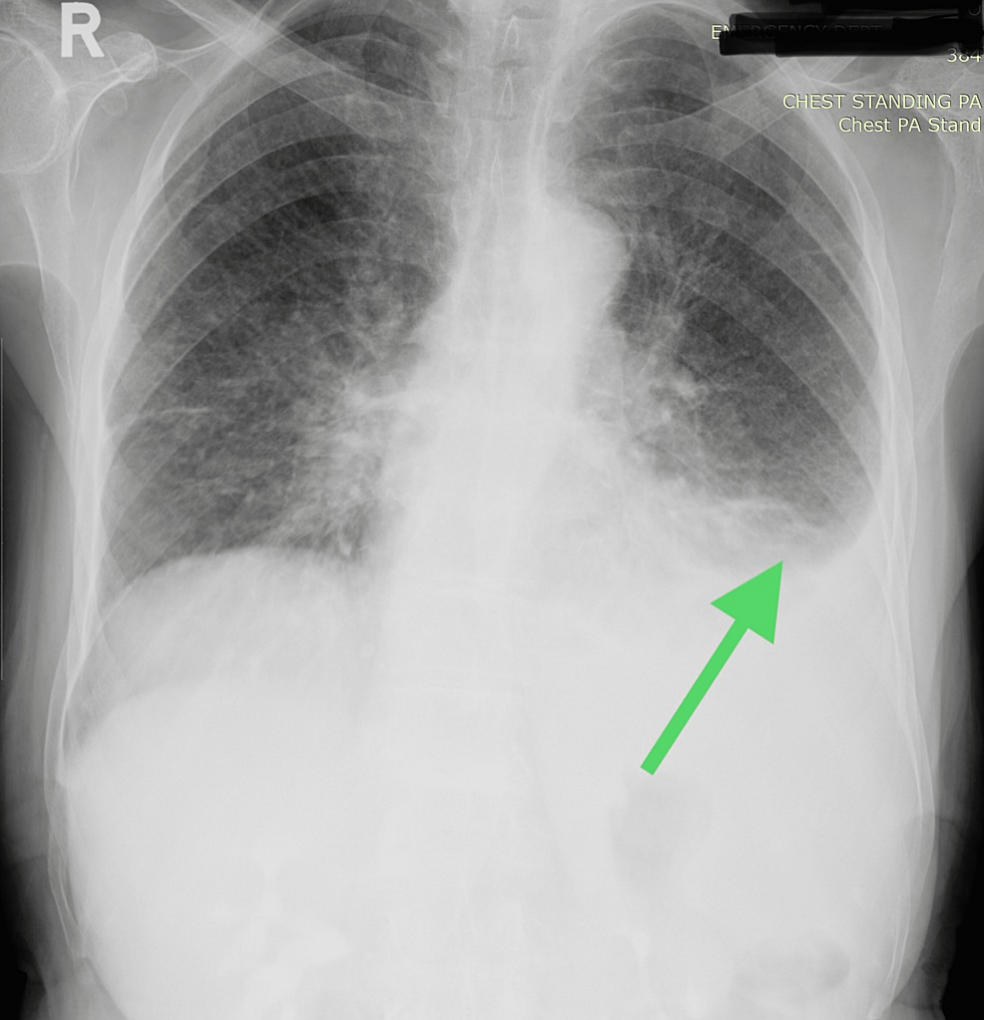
Chest X-ray; the arrow indicates left-sided pleural effusion
